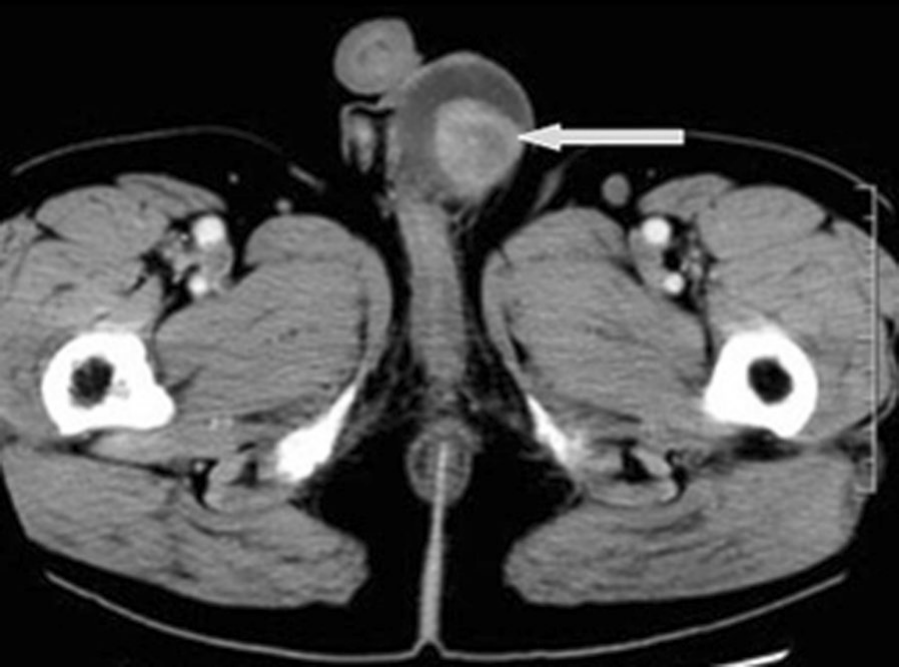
Axial CT image of scrotum revealed a solid mass measured about 3 cm × 3 cm in the left epididymis with regional extension to the testis (*White arrow*)
